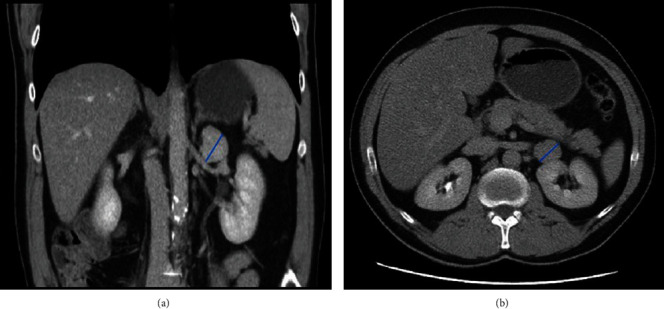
Contrast-enhanced CT revealing the tumor in the left retroperitoneal space: (a) frontal section and (b) cross-section. A blue arrow indicates the diameter of the tumor.
